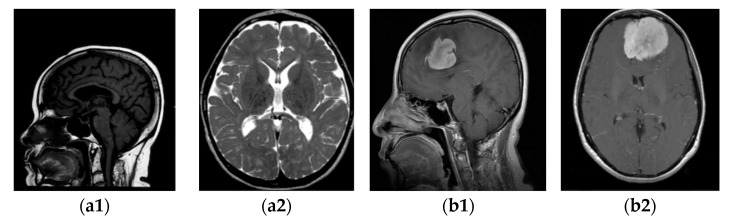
MRI images of two categories: (a1,a2) without tumour; (b1,b2) with tumour.
